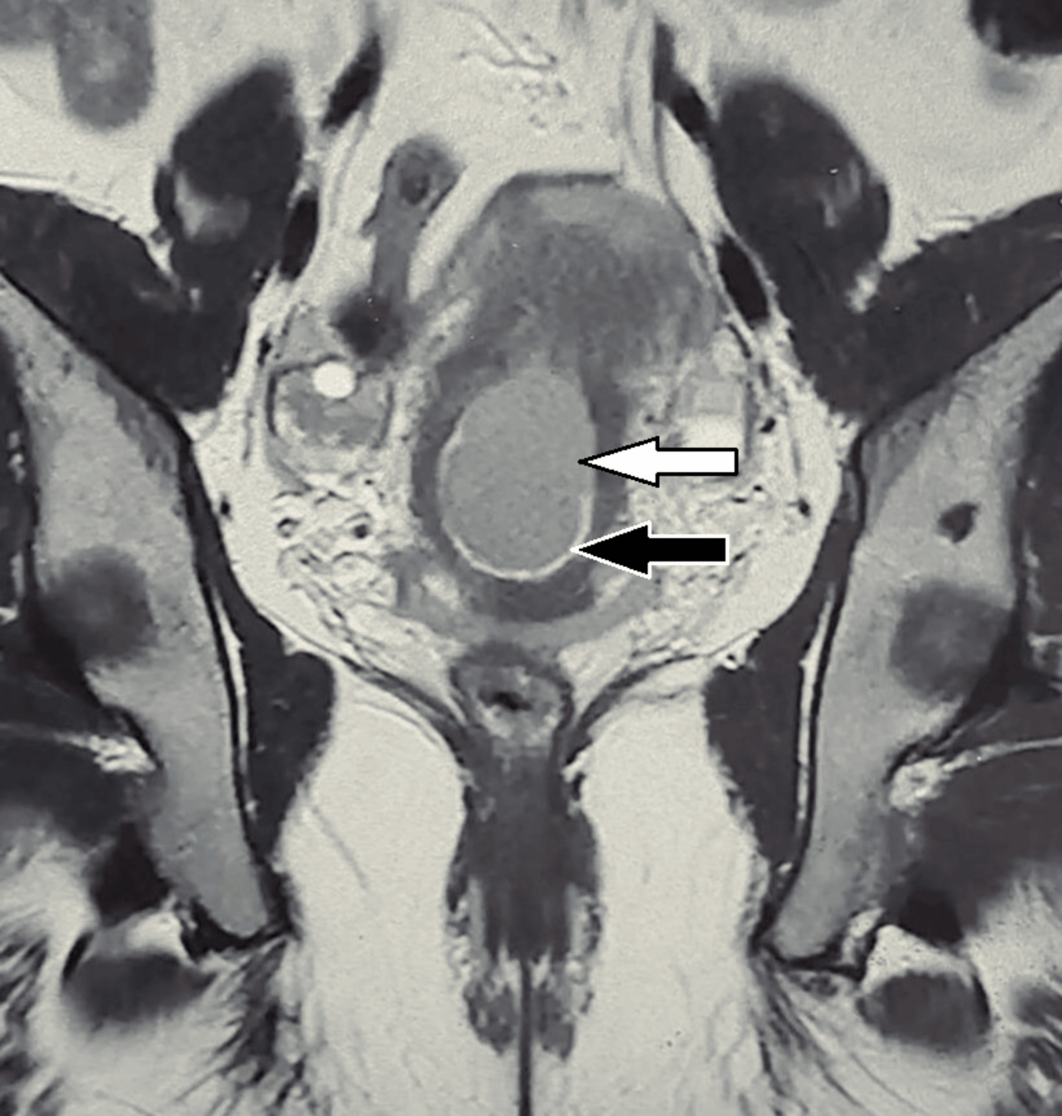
T2-weighted coronal MRI shows a hyperintense to intermediate signal intensity lesion in the lower uterine segment, endometrial cavity, and endocervical canal (black arrow). Evidence of hemorrhagic collection is seen inside the uterine cavity (white arrow). MRI: magnetic resonance imaging
